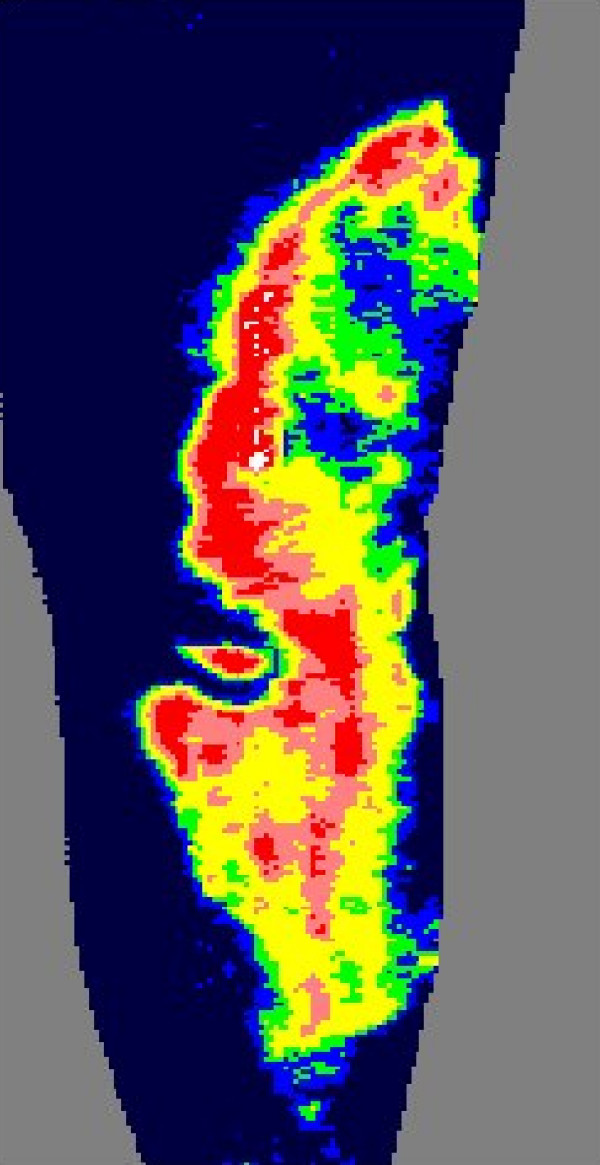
Picture of a laser-Doppler burn image.
